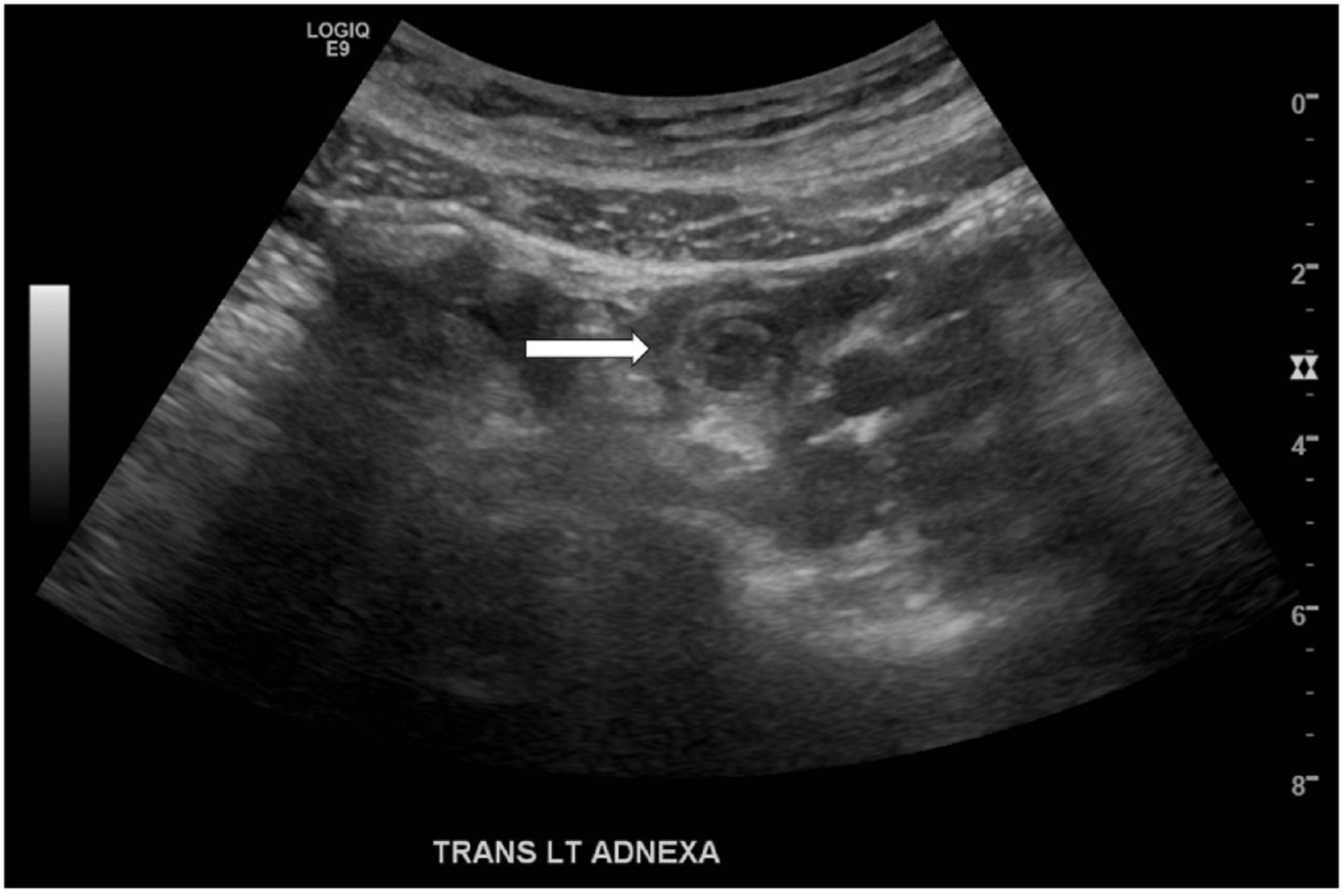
Transverse view of transabdominal pelvic ultrasound of the left adnexa revealing targetoid lesion of intussuscepted small bowel (white arrow).
